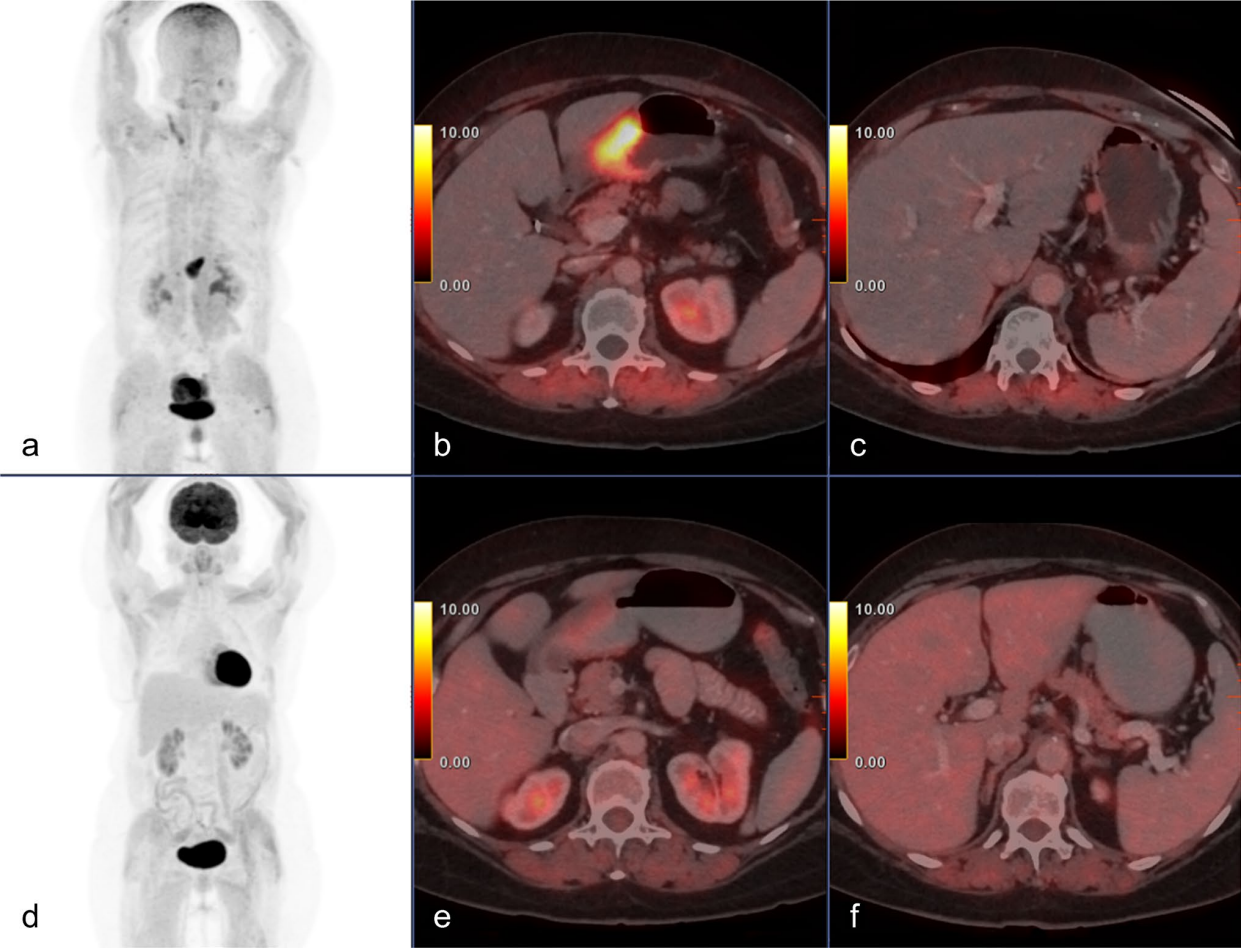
Staging FAPI (a, b, c) and FDG (d, e, f) PET/CT scans of a 65-year-old female with poorly differentiated gastric adenocarcinoma (patient #8 Table 1), showing a FAPI-positive, FDG-negative primary tumor (SUV_max_ 11.8 and 2.3, respectively) and a perigastric lymph node (SUV_max_ 0.3 and 2.3, respectively). She was treated with neoadjuvant chemotherapy and had stable disease on follow-up imaging
